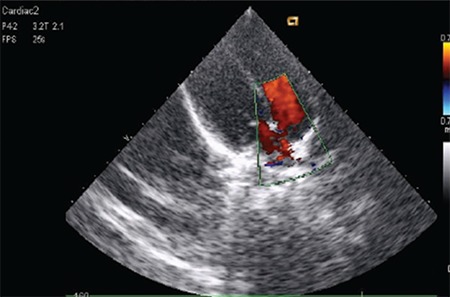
Echocardiography showing ostium secundum variant of atrial septal defect
